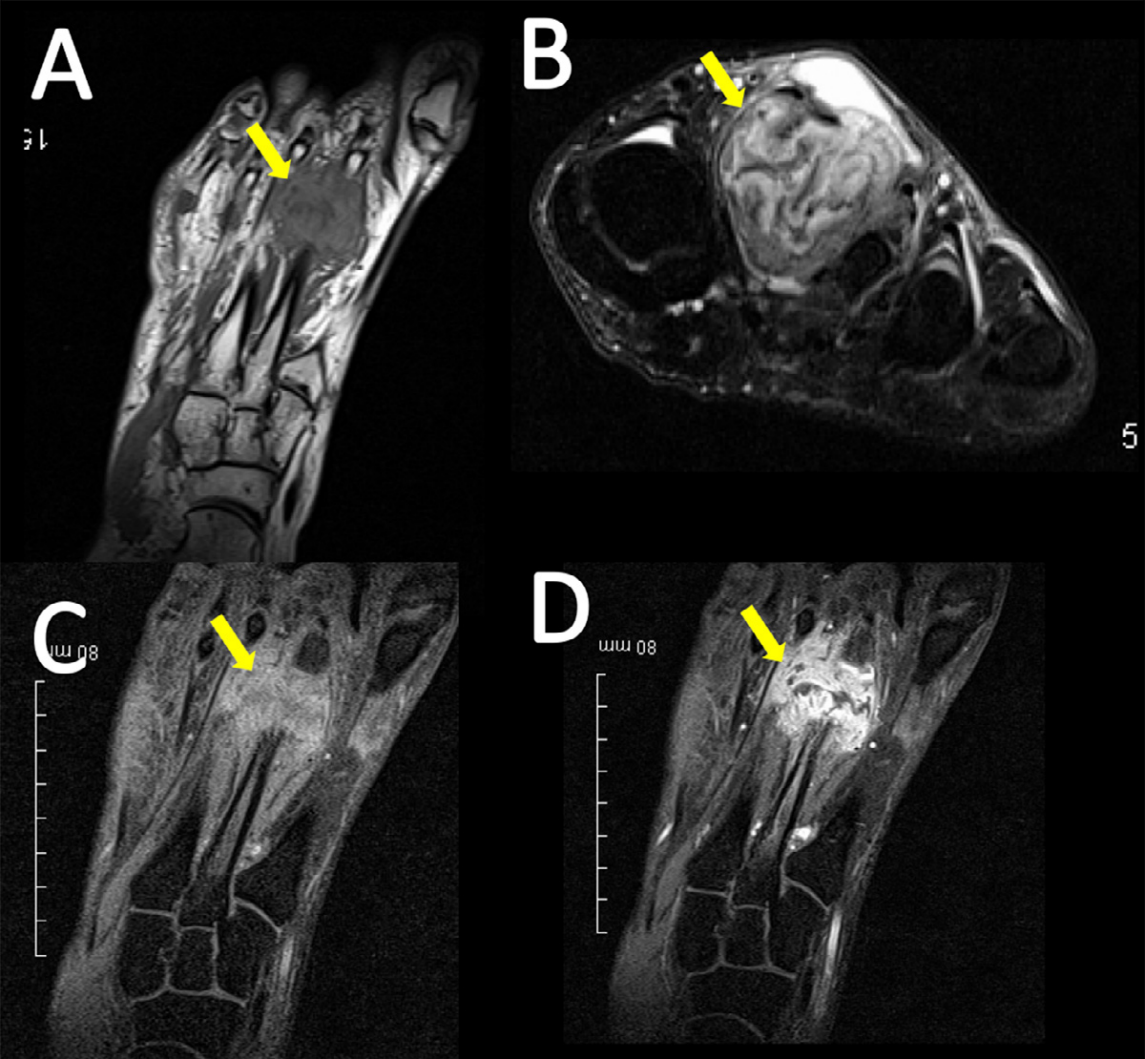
(A) Long axis T1, (B) short axis T2 STIR, (C) long access T1 STIR Pre contrast, (D) long axis T1 STIR post contrast MRI sequences of the left foot. The expansile soft tissue lesion (arrows) demonstrates T1 low signal with T2 heterogenous high signal. There is a large effusion at the dorsal aspect. There is avid post contrast enhancement within the lesion and further enhancement within the marrow of the second metatarsal. Punctate areas of signal drop out correspond to foci of calcification on CT.
